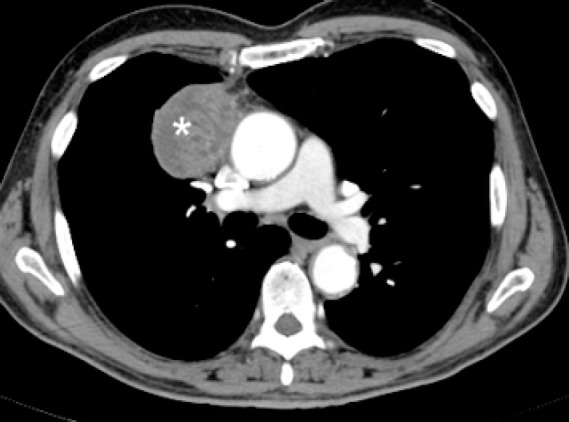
Contrast enhanced CT image of the chest of a middle aged man with an anterior mediastinal mass (asterisk) which was subsequently diagnosed to be thymoma
